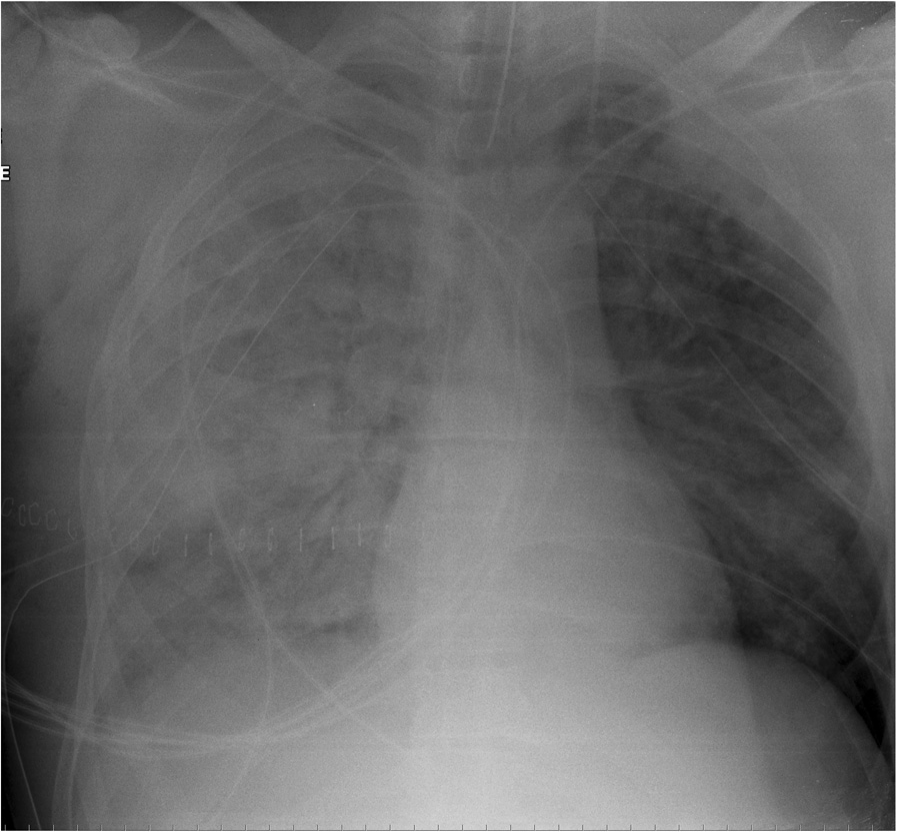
Chest X-ray taken at the admission in the intensive care unit, showing diffuse bilateral lung infiltrates and extensive airspace disease on the right lung.
